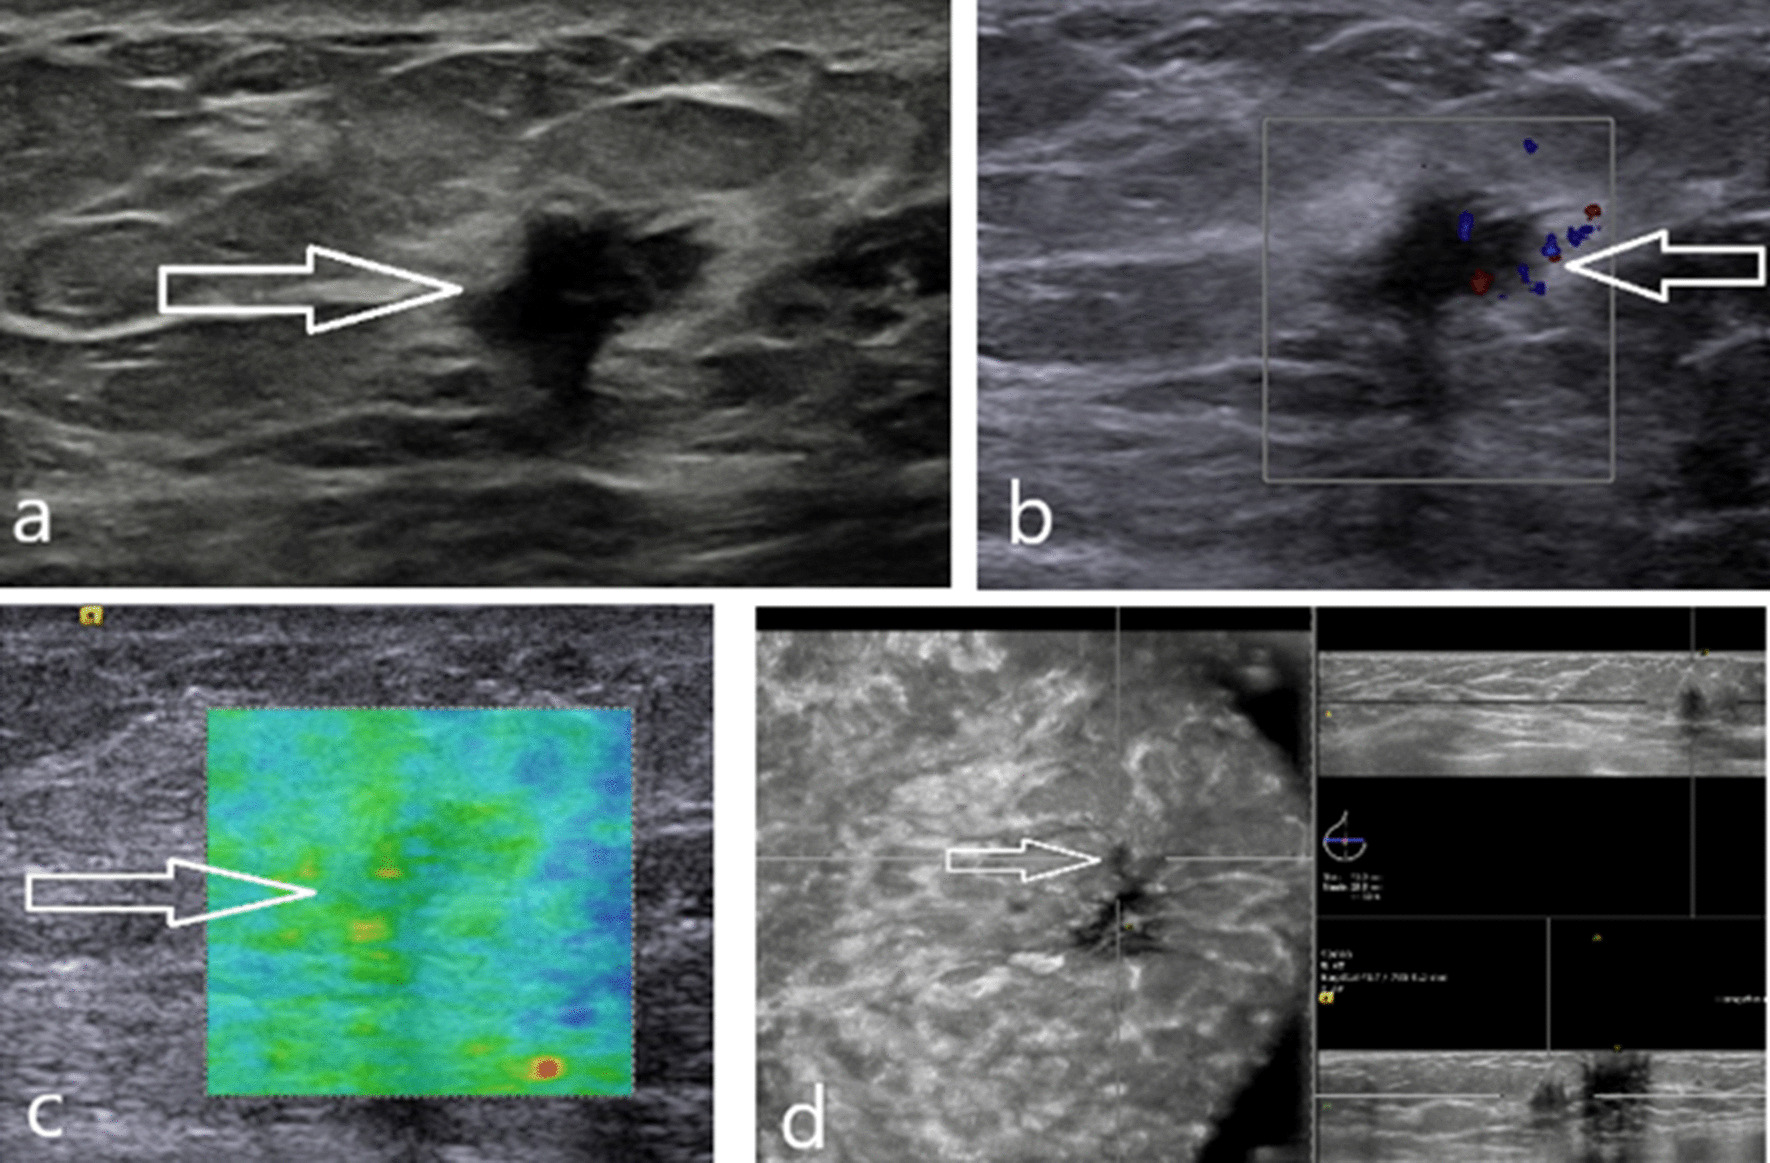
a The handheld US revealed an irregularly shaped and markedly hypoechoic nodule with angular edges and an echogenic halo surrounded by subcutaneous adipose tissue and attached to the superficial fascia of the breast (white arrows). b The surrounding adipose tissue showed an increased vascular distribution on Doppler US examination (white arrows). c Ultrasound elastography showed a Tsukuba stiffness score of 1 of the lesion (white arrows). d By ABUS, the lesion showed a retraction pattern on the coronal plane (white arrows)
